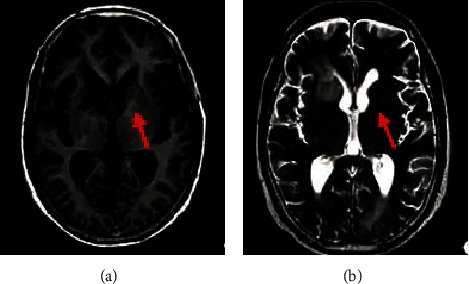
The perivascular space MRI image of a male patient aged 58 years. (a), (b) The T1WI and T2WI, respectively.
